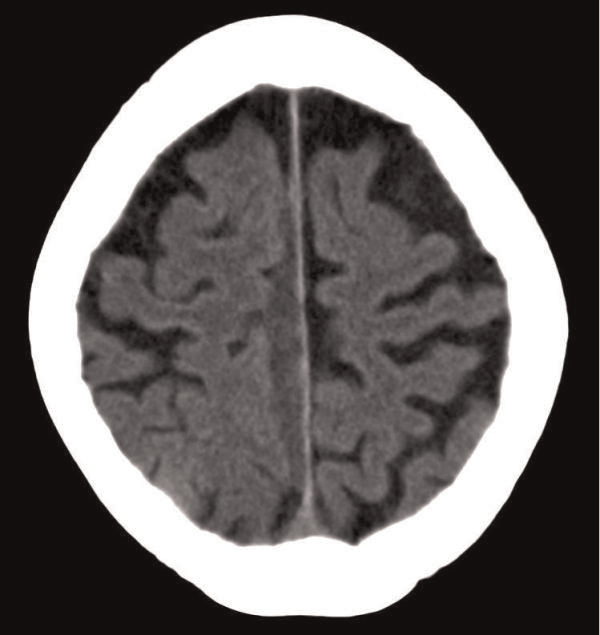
Computed tomography of the brain two weeks later shows a hypodense lesion representing an interhemispheric subdural hematoma of older age.
